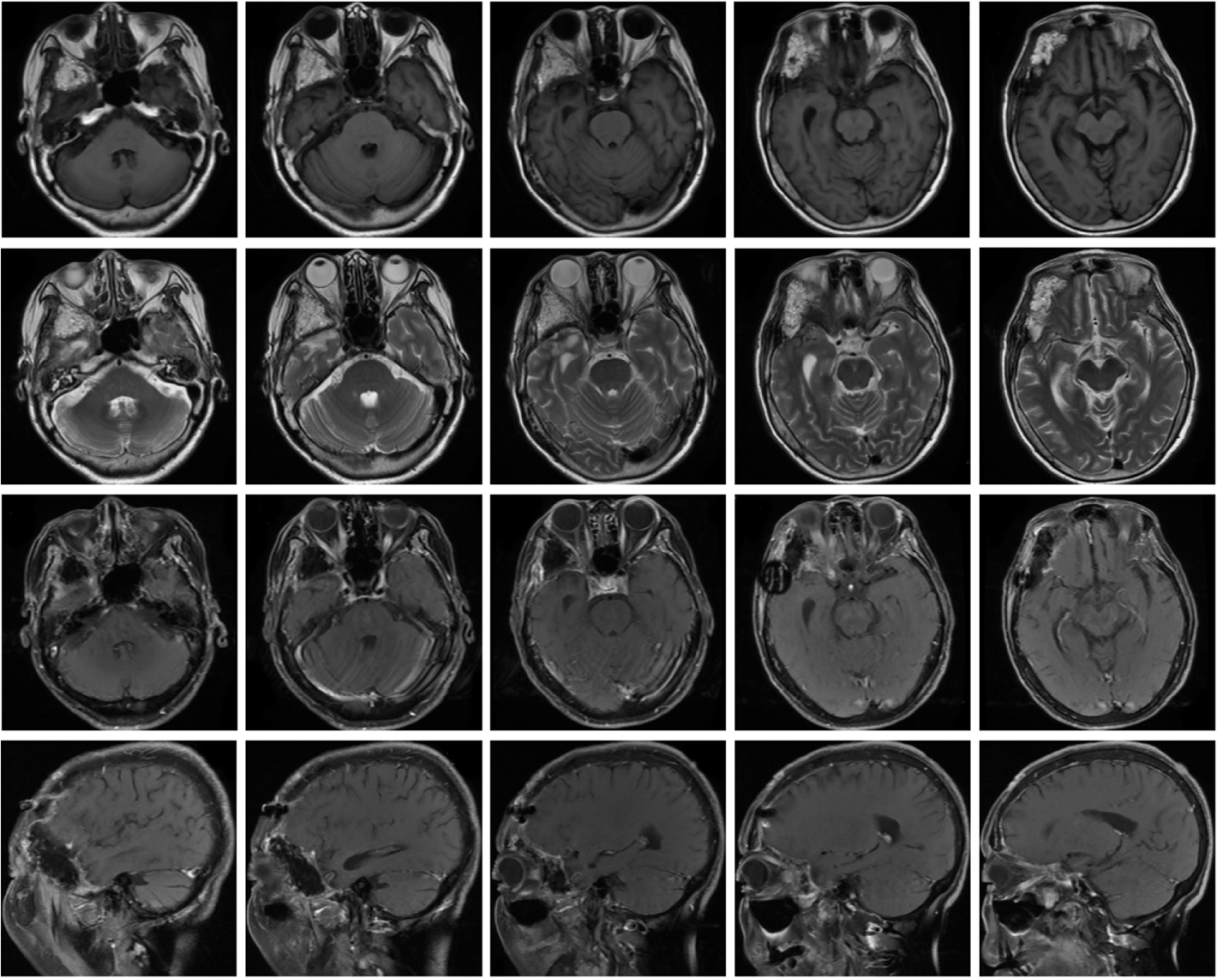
Post-operative MRI scan also showed full resection and enhanced signal from the presence of fat
